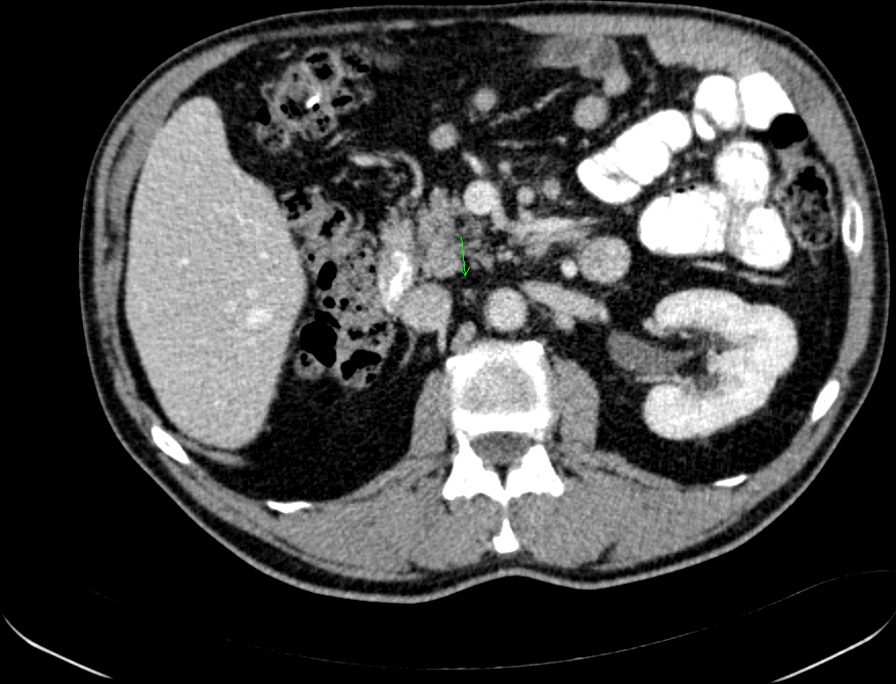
Computed tomography scan at the time of pre-planned restaging (August 9, 2018): Aortocaval retroperitoneal lymph node—the target lesion (6 mm × 5 mm)—green arrow
